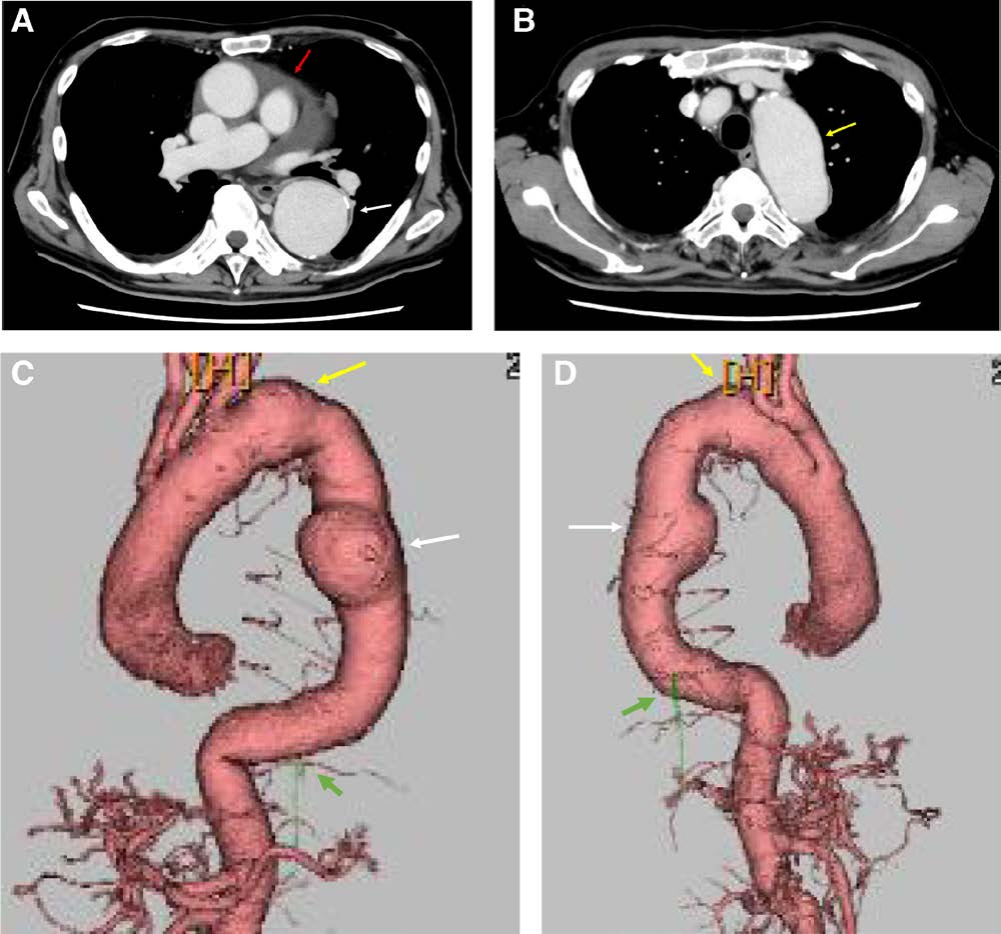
Preoperative computed tomography. (A) The red arrow indicates pericardial effusion, whereas the white arrow indicates descending aortic aneurysm. (B) The yellow arrow indicates distal aortic arch aneurysm. (C and D) The red, yellow, white, and green arrows indicate pericardial effusion, distal aortic arch aneurysm, descending aortic aneurysm, and the Adamkiewicz artery, respectively.
